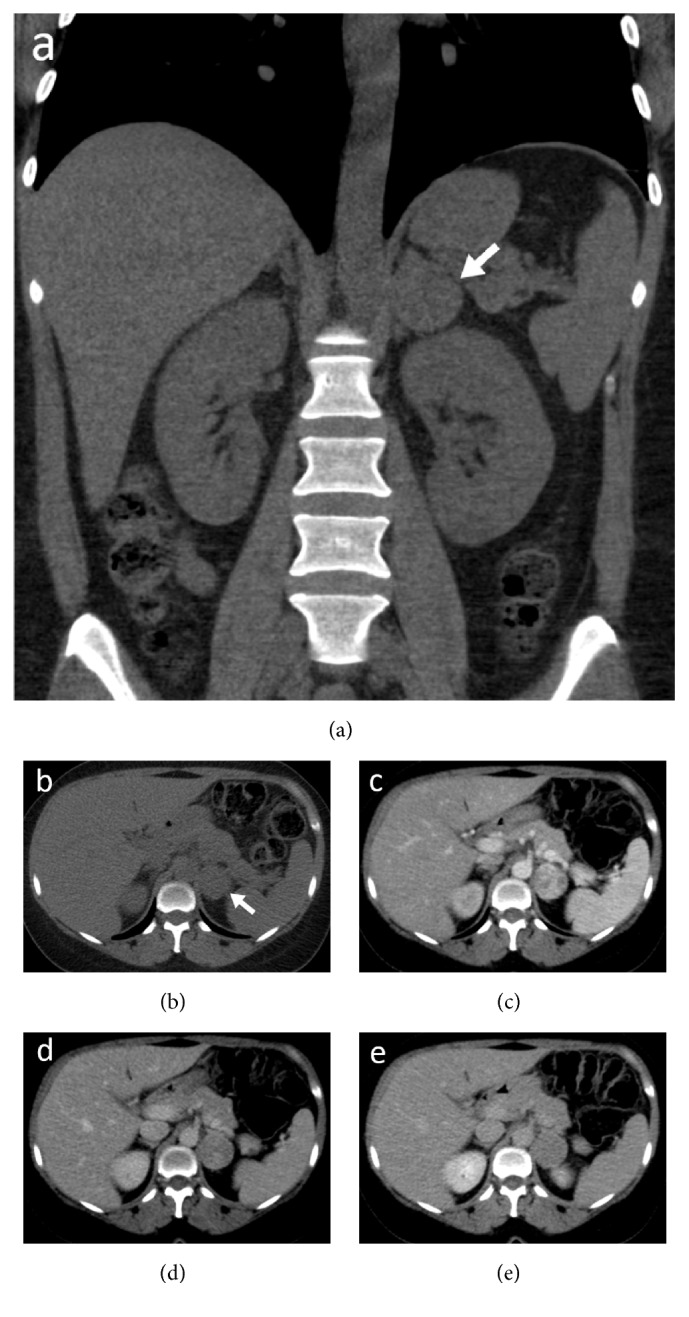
Coronal reformatted (a) and axial unenhanced CT images (b) show well-defined isodense mass in left adrenal gland measuring 3.5 cm in size. It is characterized by solid component (38 HU on unenhanced scan) (b), avid enhancement during arterial (c) and portal phases (102 HU) (d), and mild washout during delayed phase (79 HU) (e).
